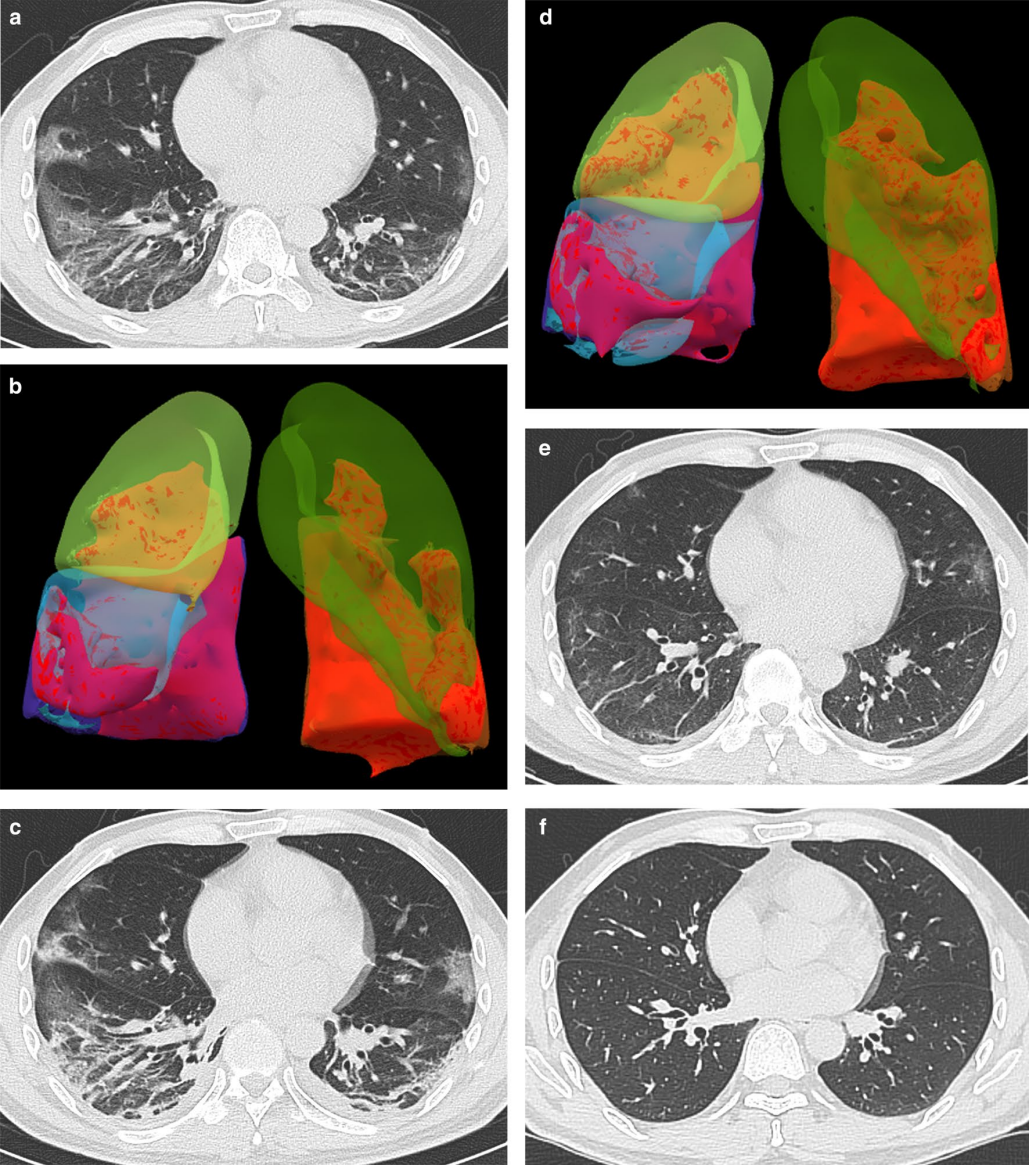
Male, 45-year-old, COVID-19. a Axial CT and (b) Volume Render (VR) Imaging. The initial CT and VR imaging obtained 7 days from onset of symptoms. The CT shows the patchy ground-glass opacity, crazy-paving pattern, irregular lines, and consolidation around the subpleural area, and pleura thickening. The VR imaging shows the lesions involve the bilateral lungs. c Axial CT and (d) VR Imaging. The peak CT and VR imaging obtained 3 days later. The CT shows that ground-glass opacity decrease, consolidation irregular lines, and pleural thickening increase, and bilateral pleura effusion occurred. The VR imaging shows the lesion size significantly increases. e Axial CT. The pre-discharge CT obtained 8 days later. The CT shows ground-glass opacity, irregular lines, and pleural thickening adhesions remain. f Axial CT. The follow-up CT obtained 34 days later shows a normal chest CT
